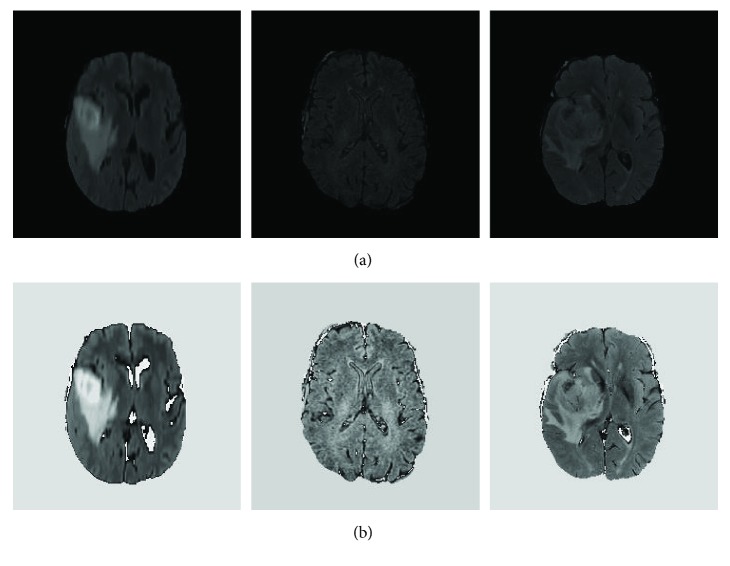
Randomly selected examples of FLAIR slices before (a) and after (b) the above-mentioned intensity normalization.
